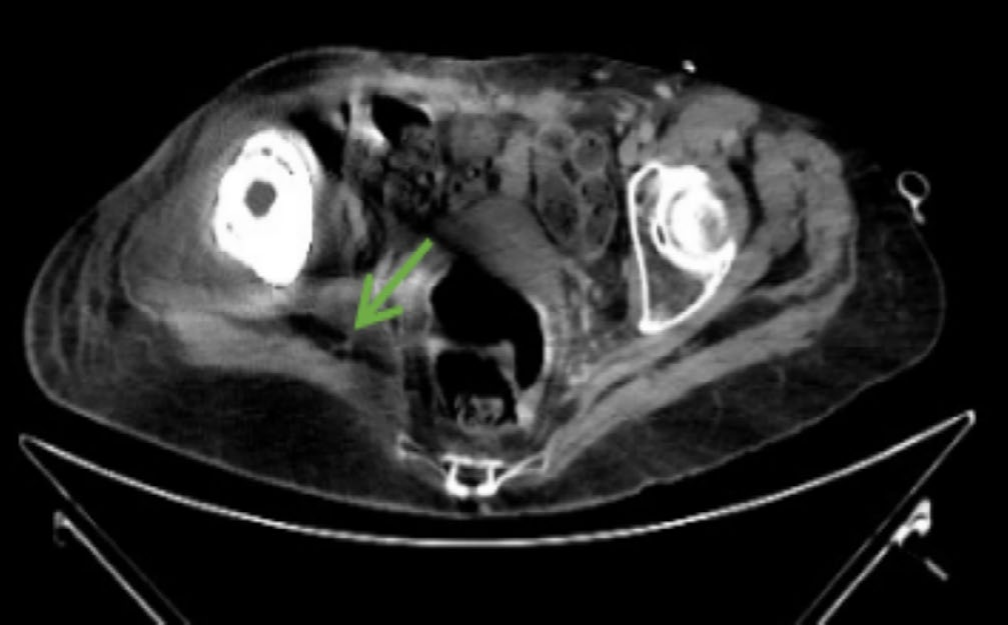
A non‐contrast CT scan showing the presence of air bubbles in the right hemipelvis.
